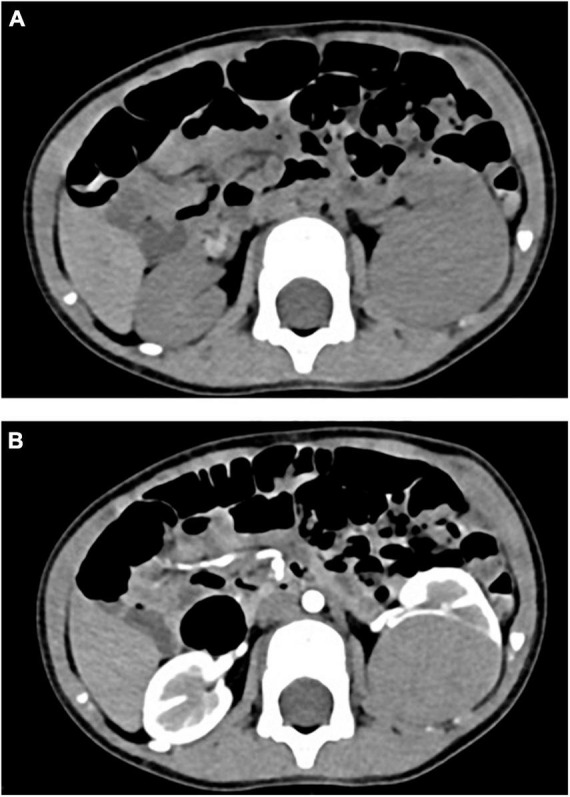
(A) CT plain scan before chemotherapy: CT value was 46 HU. (B) CT enhanced before chemotherapy: the third stage showed obvious uneven enhancement, with CT values of about 58 HU, 98 HU, and 133 HU, respectively.
